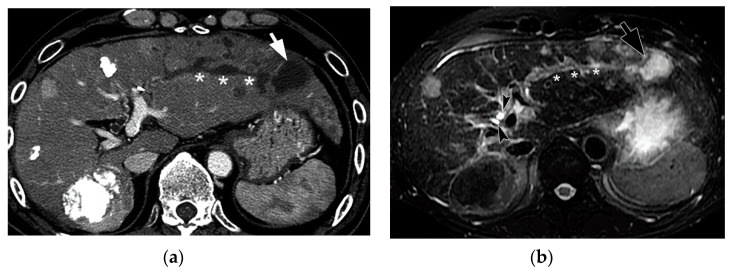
Comparison between CT and T2-weighted MRI findings of BDN. (a) On contrast-enhanced CT, a hypodense irregular tubular structure (*) is observed in the left lateral segment of the liver. Although this tube-like structure looks like irregularly dilated intrahepatic bile ducts, it is BDN. Biloma formation (arrow) is observed at the peripheral portion of the lateral segment which is connected to BDN. On the hepatic hilum, slightly dilated right and left hepatic ducts are observed (arrowheads). (b) On T2-weighted MRI, BDN (*) and biloma (arrow) on the left lateral lobe of the liver do not show marked hyperintensity like the hepatic ducts (arrowheads), but instead show slight hyperintensity. This finding suggests that BDN and biloma are not pure fluid-filled spaces, but “necrotic tissue”. (Figure 13a is same figure as Figure 9. It is presented for comparison with Figure 13b).
